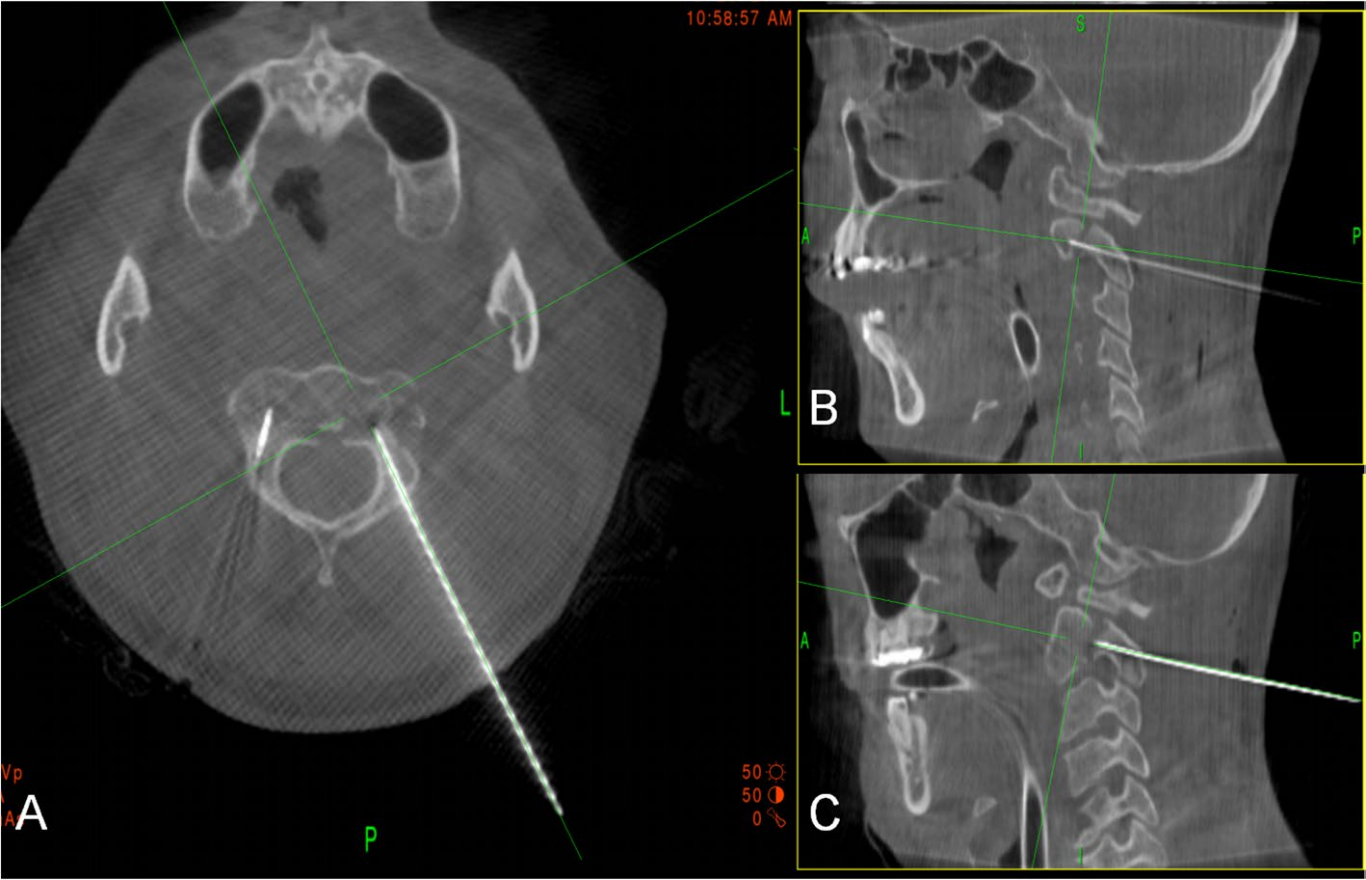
Intraoperative computed tomographic (CT) scans showing right-sided K-wire in axial and sagittal planes in C2 pedicle (A, B). Intraoperative CT scans showing left-sided K-wire in axial and sagittal planes in C2 pedicles (A, C)
